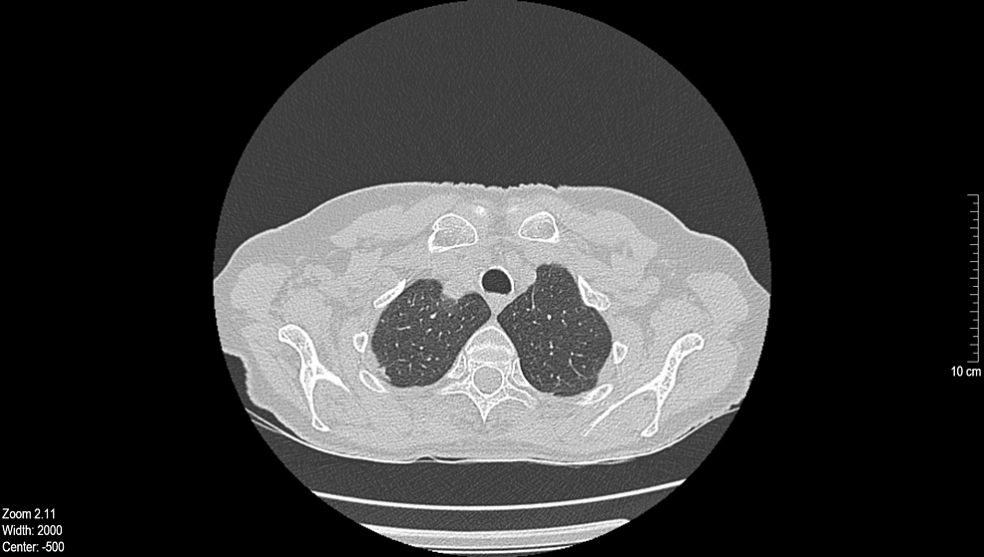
Chest CT of the patient showing a subpleural nodule
